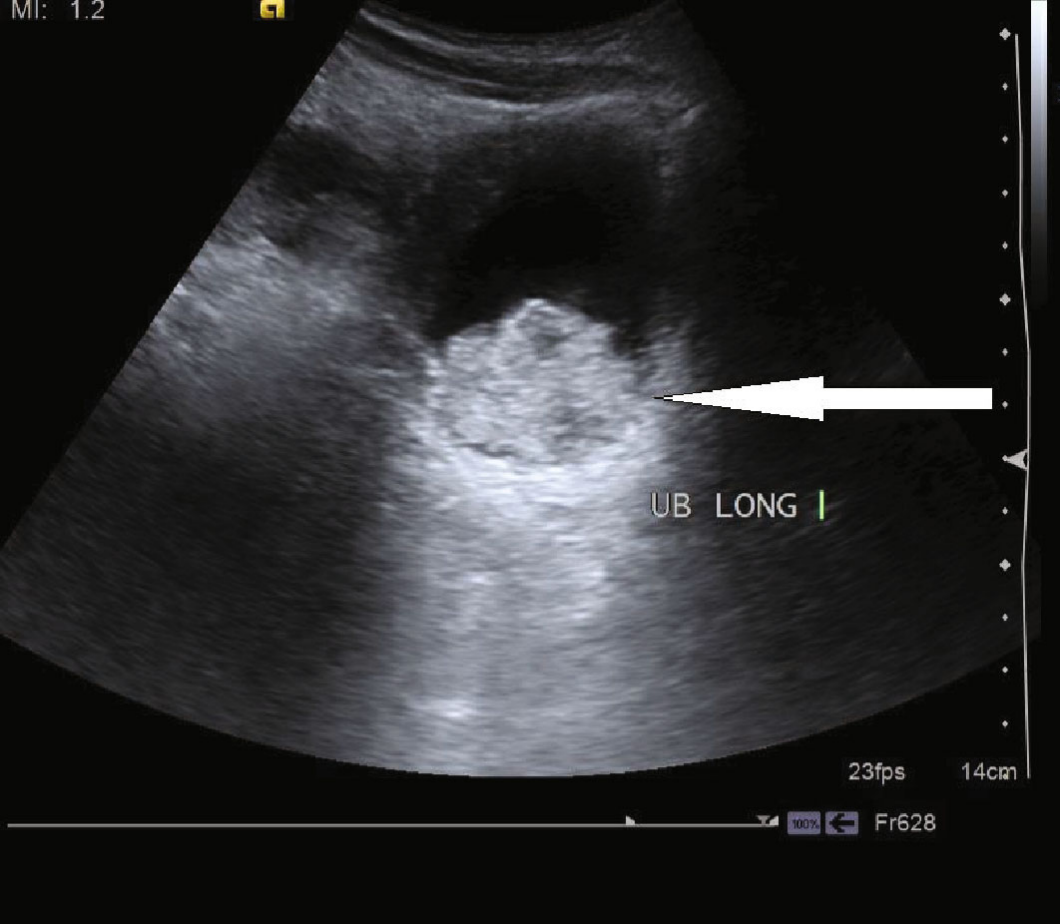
Ultrasonography showing hyperechogenic contents in the urinary bladder and thickened bladder wall.
